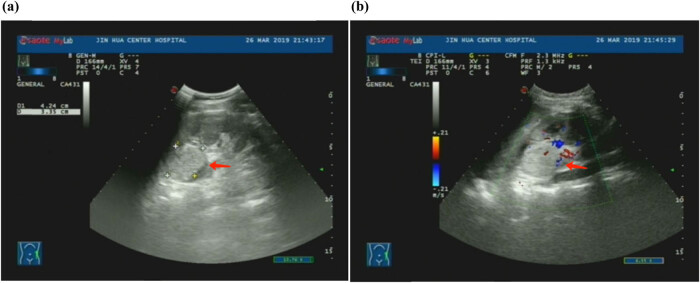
(a) Ultrasound showing a high echogenic area with internal uneven echo in the upper pole of the left kidney. (b) Ultrasound showing blood flow signal inside the echogenicity.
